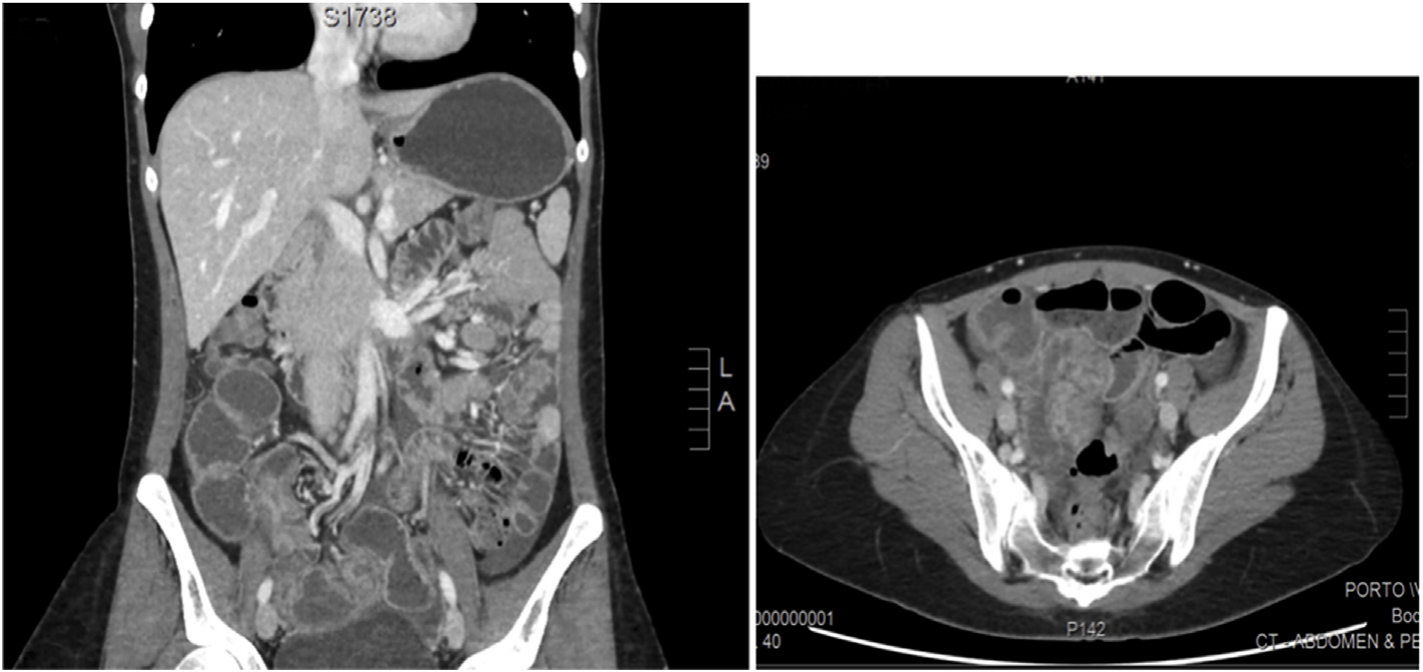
CT scan of the abdomen showing dilatation of the terminal ileum due to a compressing effect by mass causing significant stenosis and partial small bowel obstruction.
